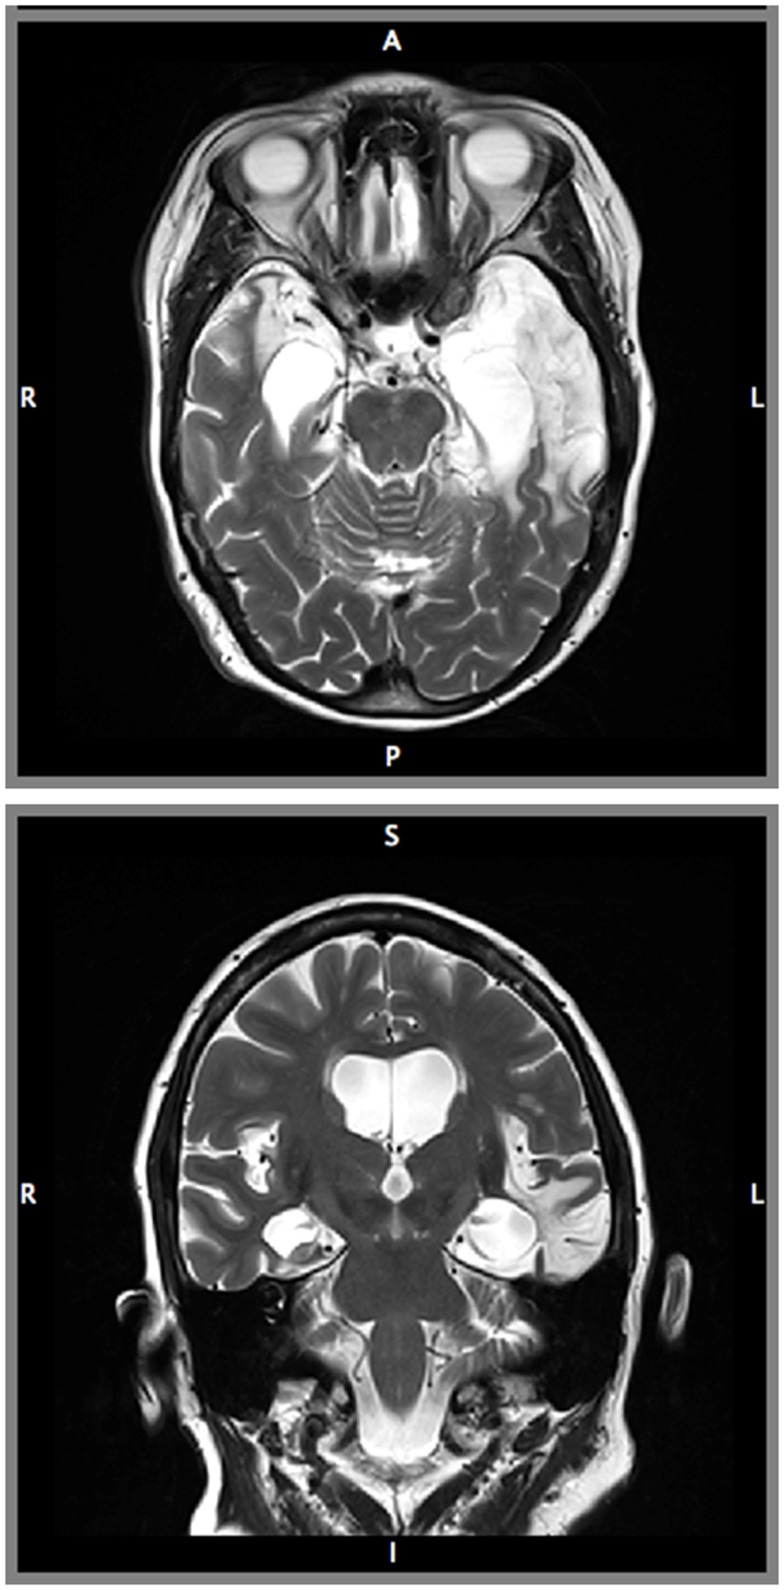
Magnetic resonance images of patient LSJ’s brain: axial (Top) and coronal (Bottom) view.
